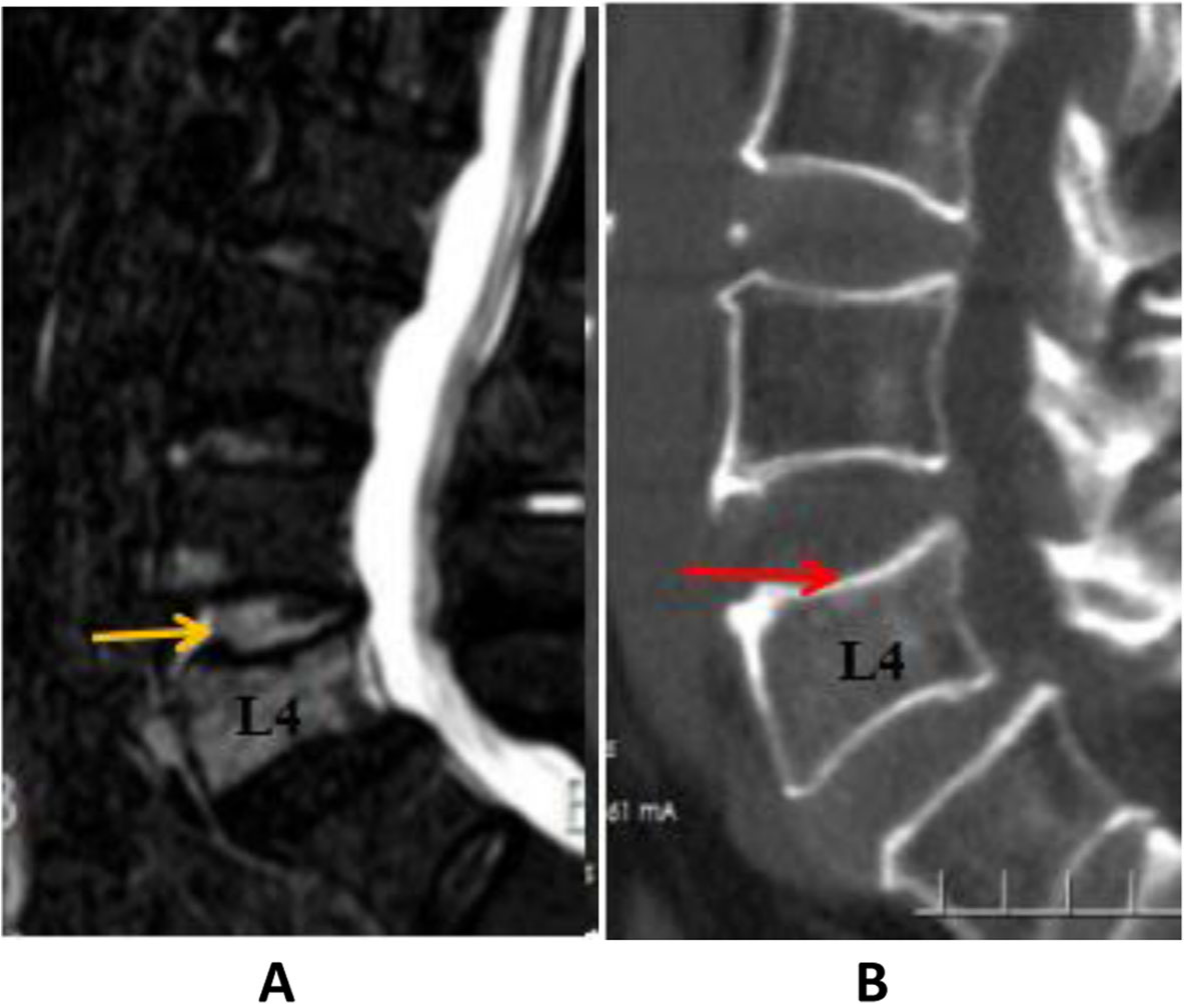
A 68-year-old man with OVF at level L4. Panel a is an MRI STIR image. The yellow arrow indicates a diffuse high-intensity signal in the IVD on the cranial side of the fractured vertebral body whose signal intensity is close to that of cerebrospinal fluid. Panel b is a CT image of the fractured vertebral body. The red arrow indicates that the upper endplate of the L4 vertebral body is intact. This is a grade 1 EDC injury as delineated in this study
